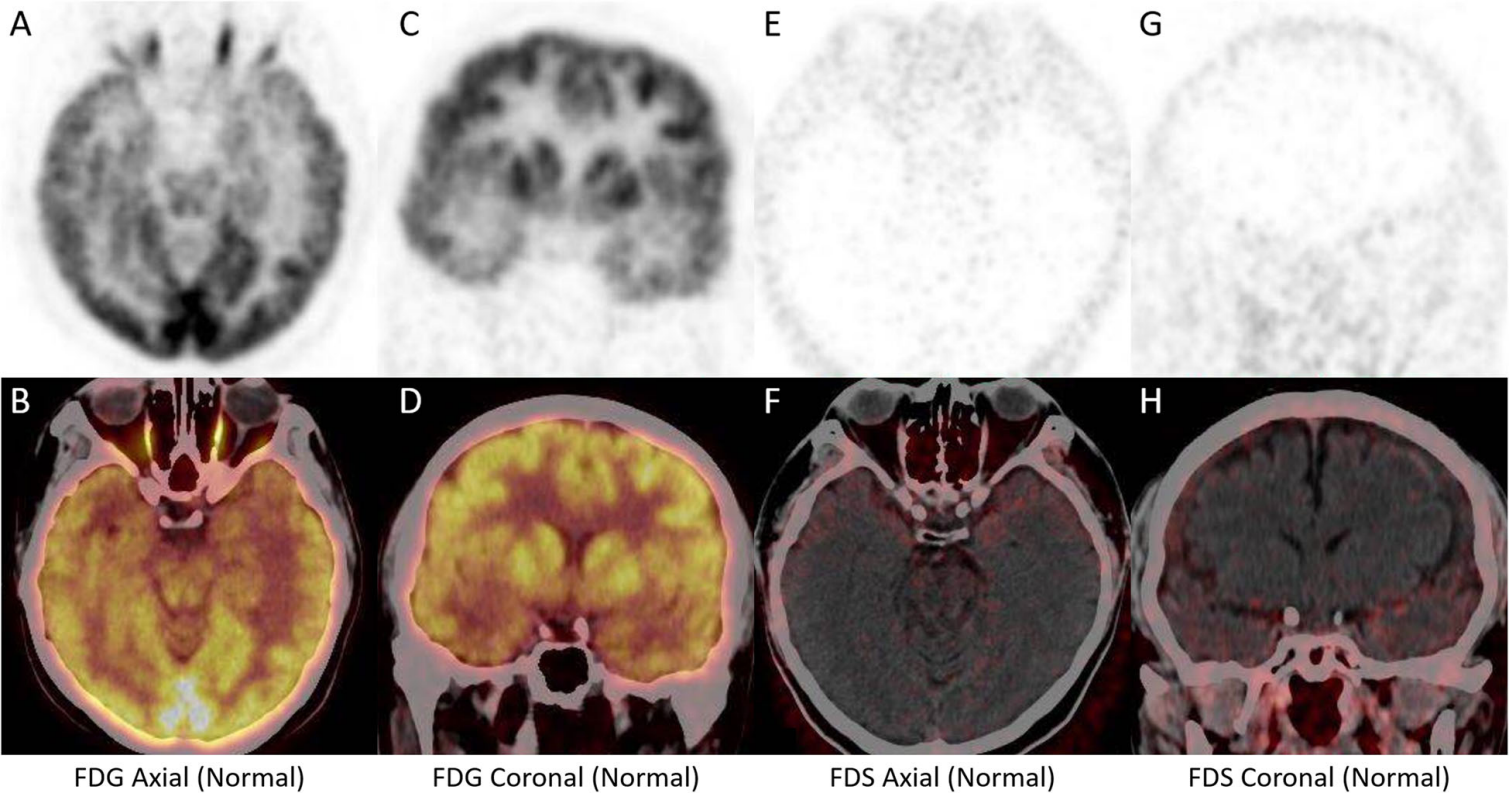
FDG and FDS activity in a patient with a normal pituitary. a-d. Both the pituitary and pituitary stalks did not display FDG activity. e-h. FDS was also not absorbed in normal pituitary and pituitary stalks
